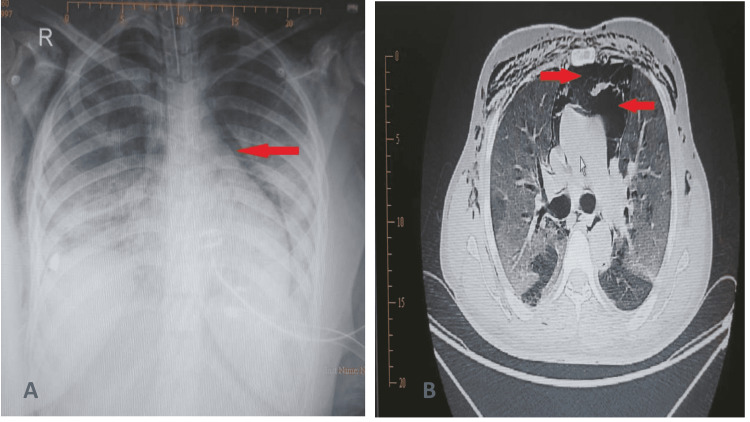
Pneumomediastinum on (A) chest radiograph and (B) axial chest computed tomography The red arrows point to the pneumomediastinum.
